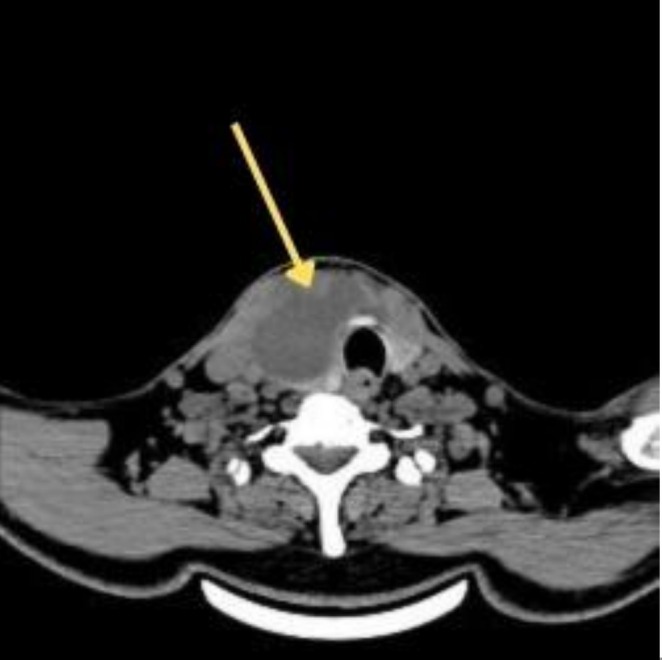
Contrast‐enhanced computed tomography (CECT) of the neck (axial section) showing a well‐defined thick‐walled hypodense lesion with peripheral enhancement involving the right lobe and isthmus of the thyroid gland (arrow), suggestive of thyroid abscess with surrounding inflammatory changes.
